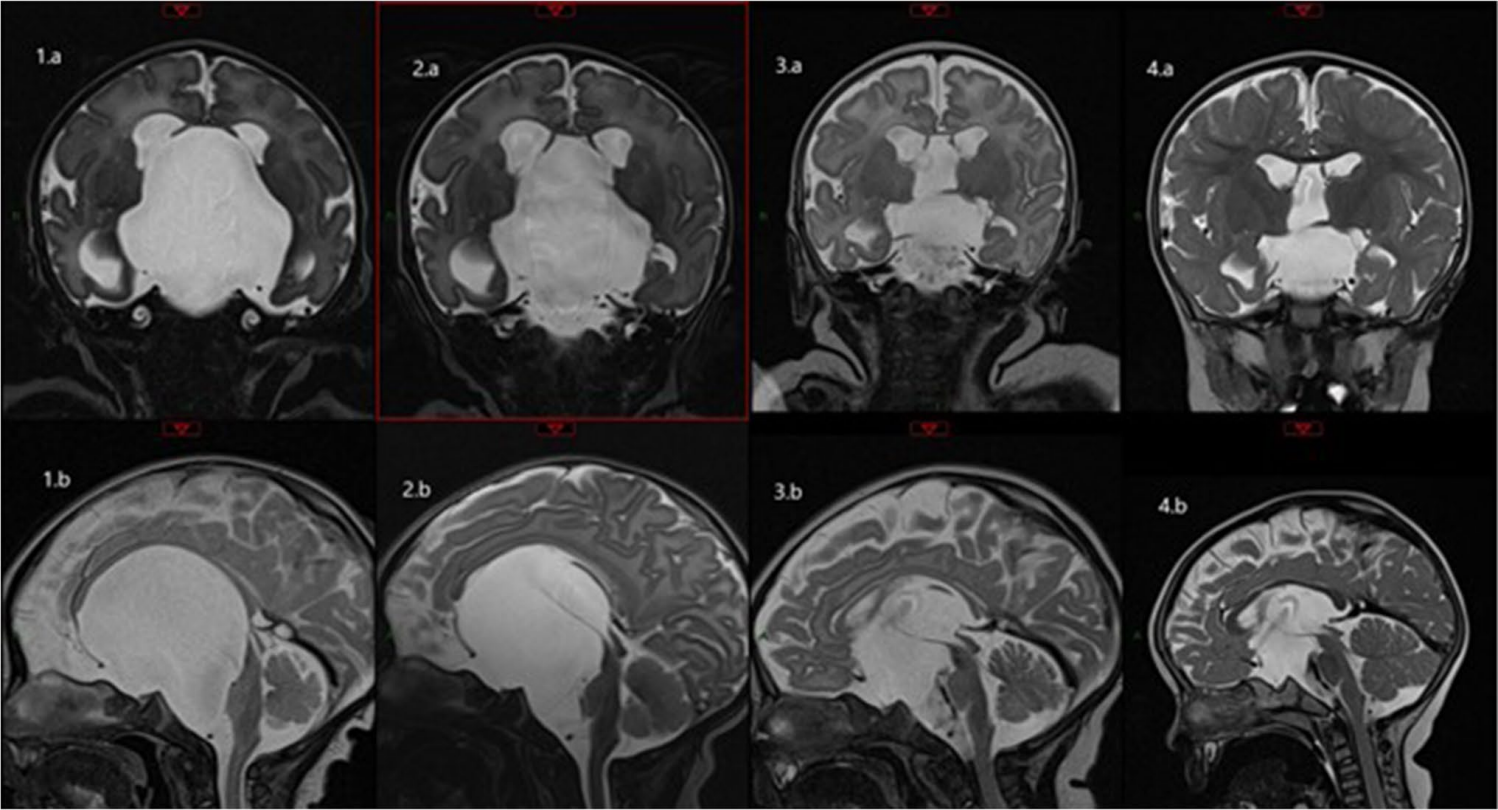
Sequential MRI demonstrating reduction in size of arachnoid cyst and ventricles. Note flow void from dorsal aspect of cyst into third ventricle. Left to right: 1. MRI at birth (a. coronal, b. sagittal), 2. MRI at D1 post procedure (a. coronal, b. sagittal), 3. MRI at 6 weeks post procedure (a. coronal, b. sagittal), 4. MRI at 14 months post procedure (a. coronal, b. sagittal)
